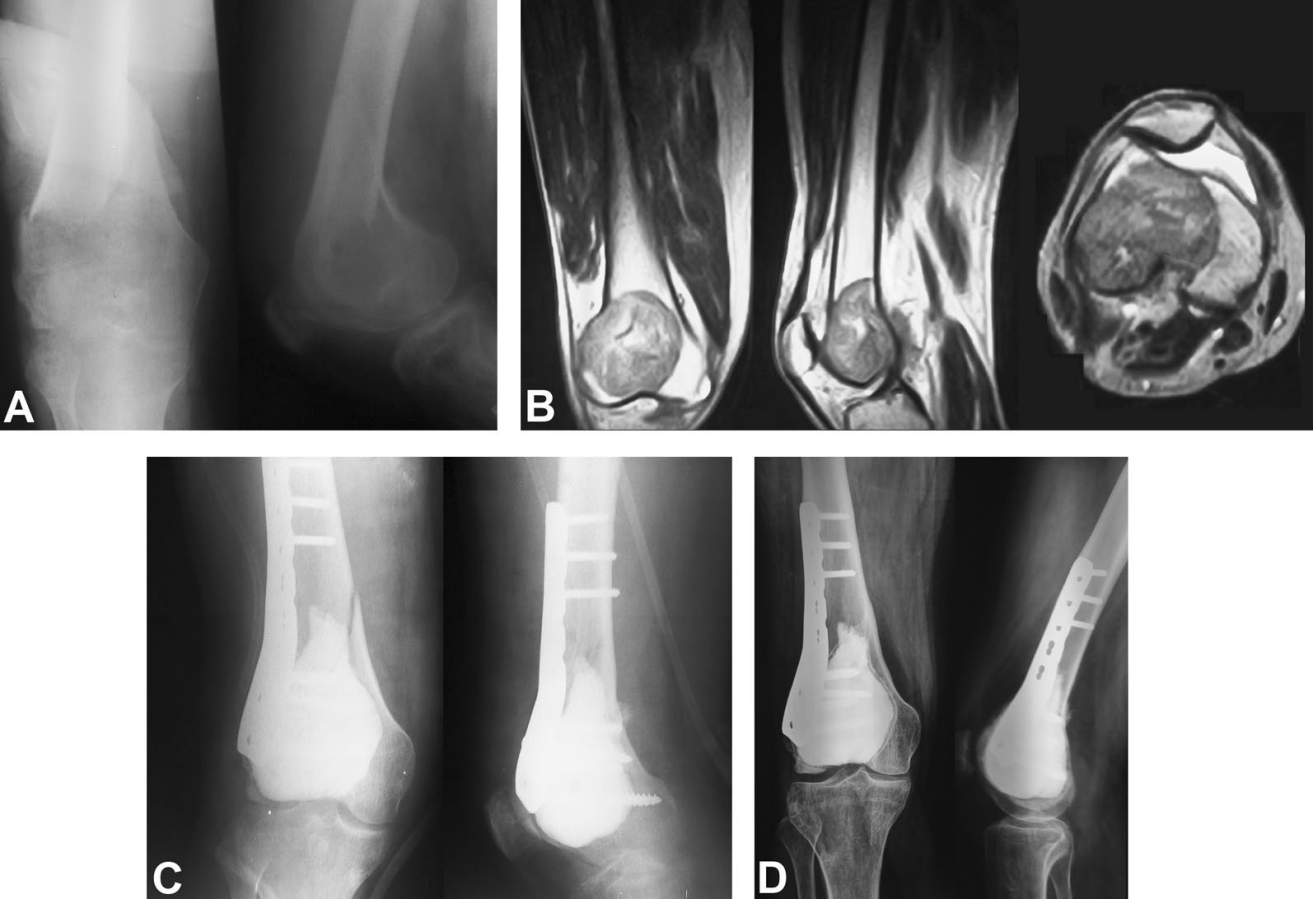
A 29-year-old male with giant cell tumor and pathologic fracture of the distal femur treated with curettage and cementation with distal femur plate fixation. A Preoperative AP and lateral X-rays. B Preoperative coronal, sagittal, and axial MRI cuts. C Immediate postoperative AP and lateral X-rays. D Ten-year follow-up AP and lateral X-rays with KL grade 1
